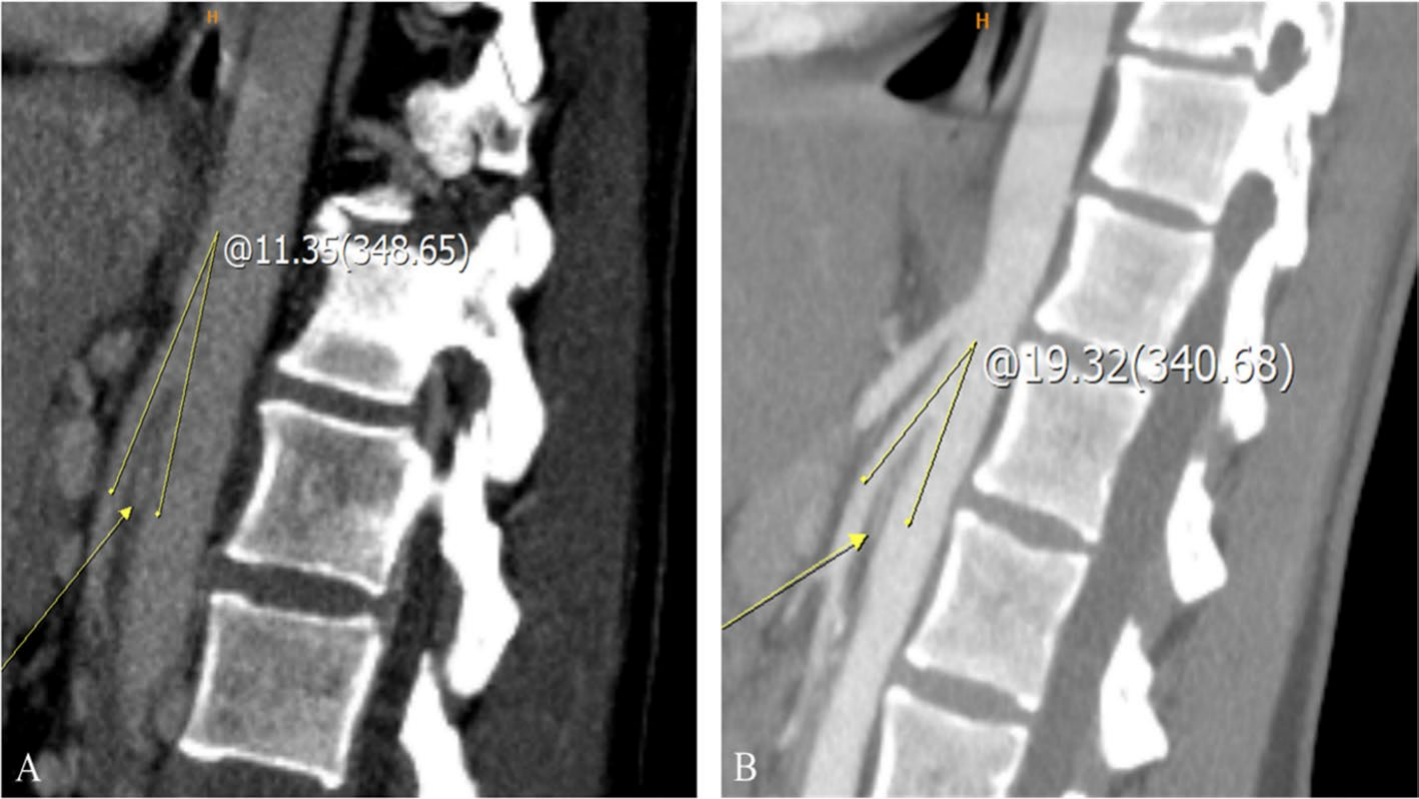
CT measurements of AMA in the sagittal plane: A portal venous phase—11° AMA indicative of NCS (yellow arrow depicting severely compressed LRV) incidentally detected in a 37-year-old female patient during staging for malignant disease; B arterial phase—19° AMA indicative of NCS (yellow arrow depicting severely compressed LRV) detected in a 17-year-old female patient who presented with a long-lasting non-specific abdominal pain
